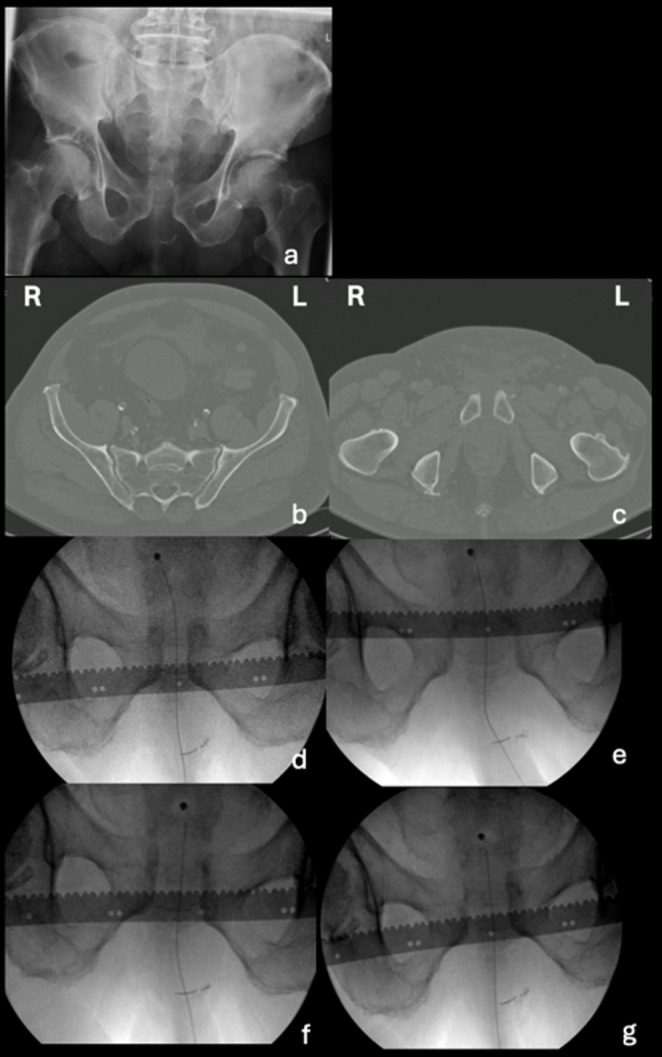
Case 10, a AP static radiograph, b CT scan showing the sacroiliac joint, c CT scan showing pubic symphysis, d AP EUA image in endorotation stress (lateral compression), e AP EUA image in exorotation stress (frog position), f AP EUA image in push right leg/ pull left leg stress (rotational instability), g AP EUA image in push left leg/ pull right leg stress (rotational instability)
